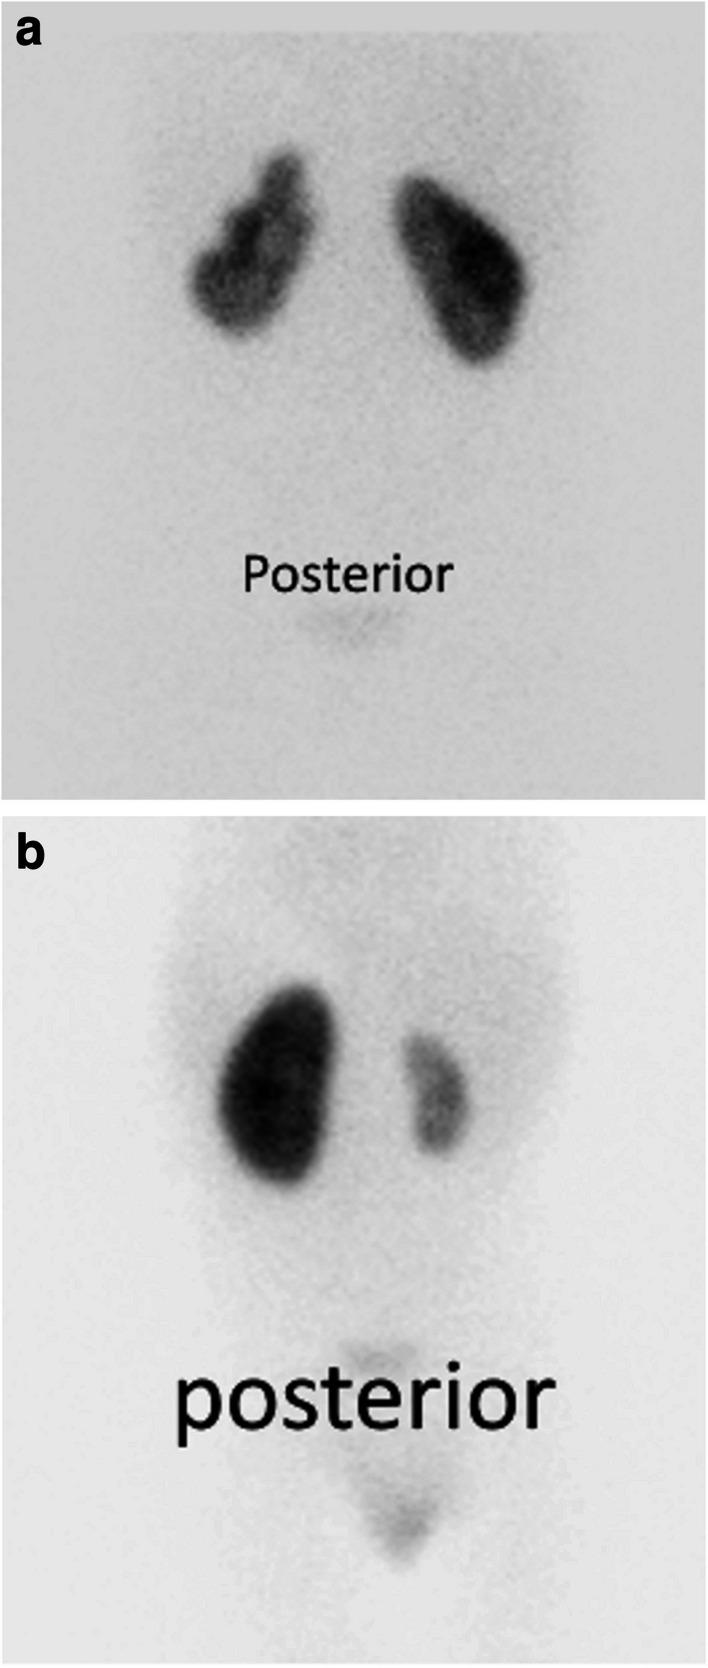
a Focal defects. Seven-year-old girl with recurrent febrile UTIs. Most recent infection occurred 8 months prior to the DMSA scan. A posterior view image shows wedge-shaped defects in the lateral aspect of both upper and lower poles of the left kidney. The defects distort the kidney contour and cause parenchymal volume loss. b Diffuse inhomogeneity. Fifteen-month-old girl with a febrile UTI 8 months prior to scintigraphy. Kidney ultrasound reported a small right kidney. A posterior view image shows a small right kidney with a functional length of 4.5 cm and a relative function of 13%. Parenchymal uptake is reduced and inhomogeneous but the renal contour is preserved
